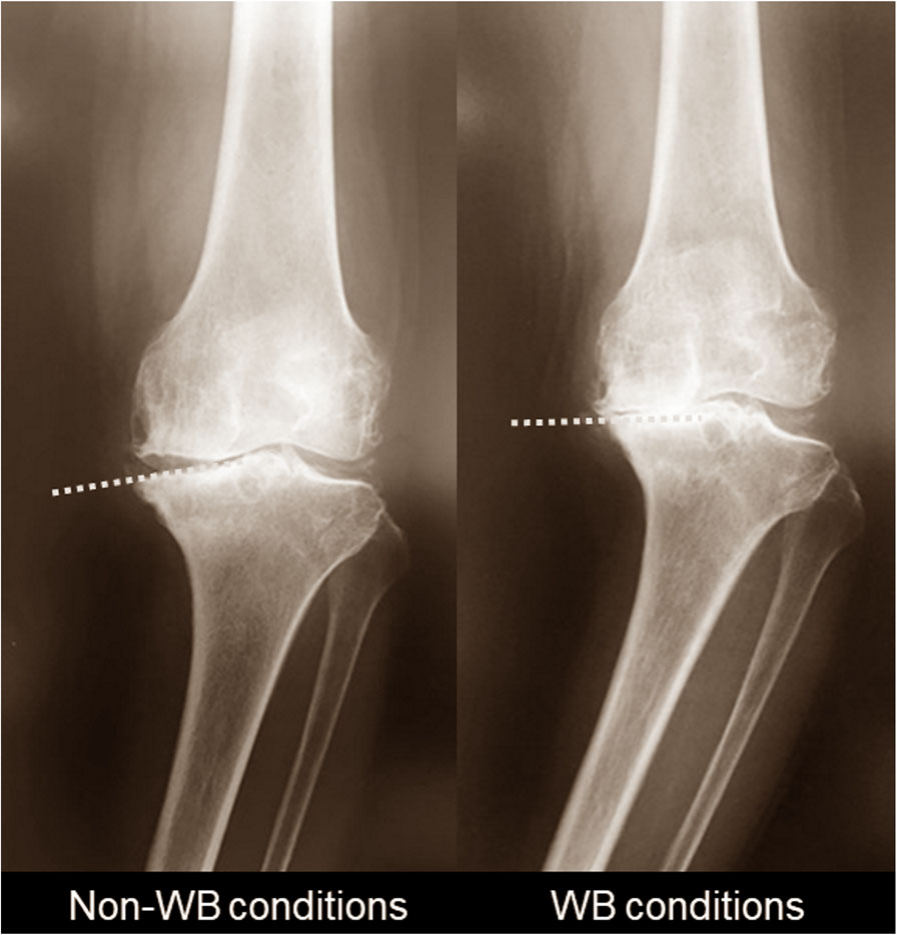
Knee radiographs in advanced knee OA under non-WB and WB conditions. An articular surface in the MCT under WB conditions was aligned parallel to the ground, compared to that under non-WB conditions
